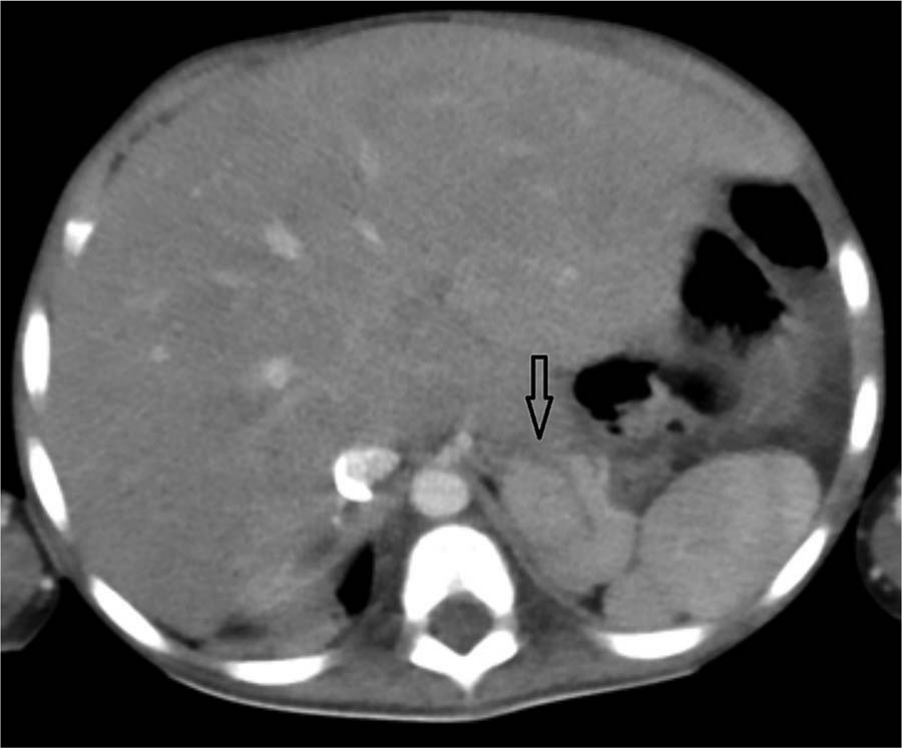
Axial abdominal CT showing central liver and polysplenism, compatible with left isomerism.
